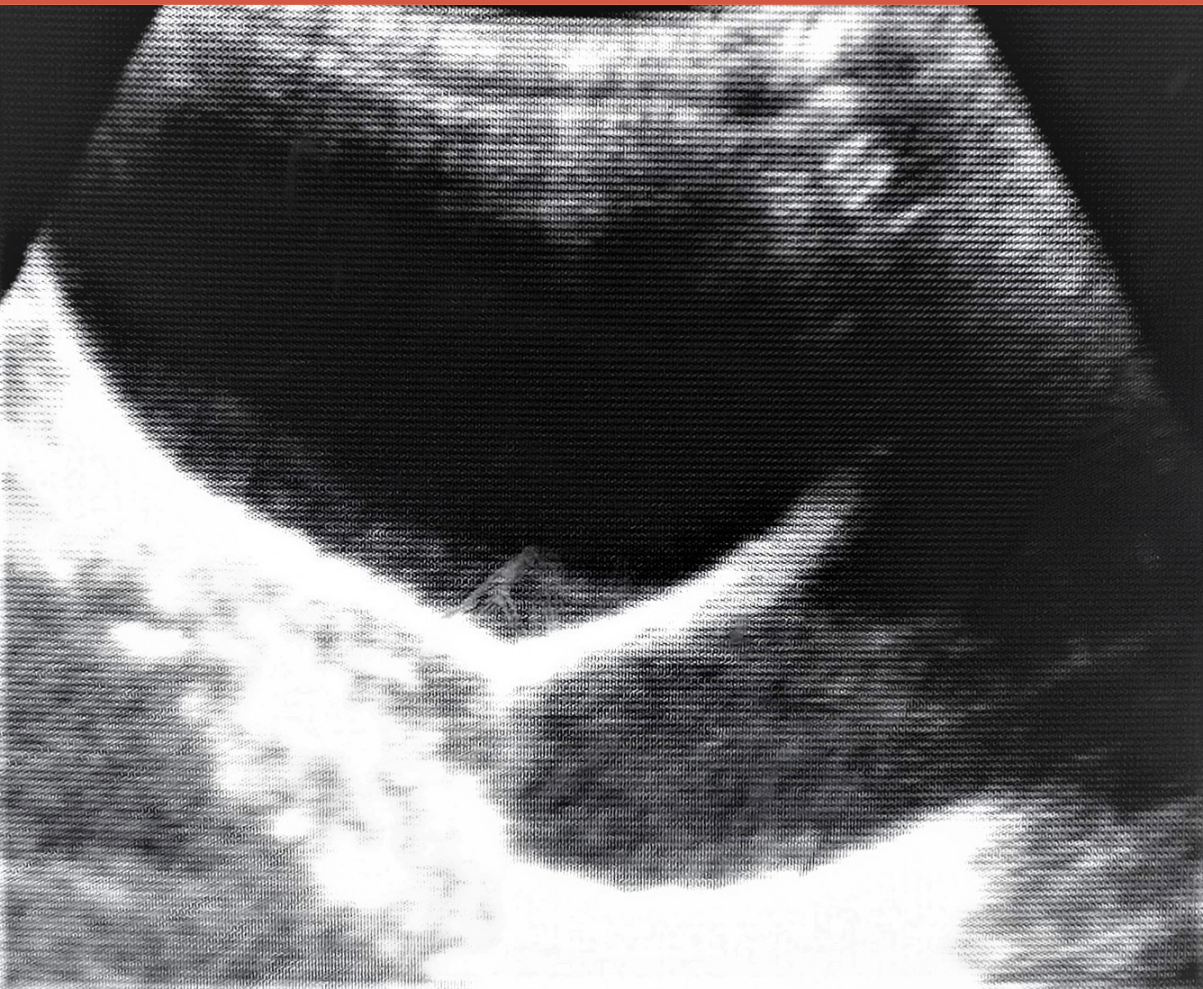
Image échographique avant la miction, montrant une réplétion vésicale et une autre image liquidienne Ultrasound image obtained before voiding, showing full bladder and another fluid image
